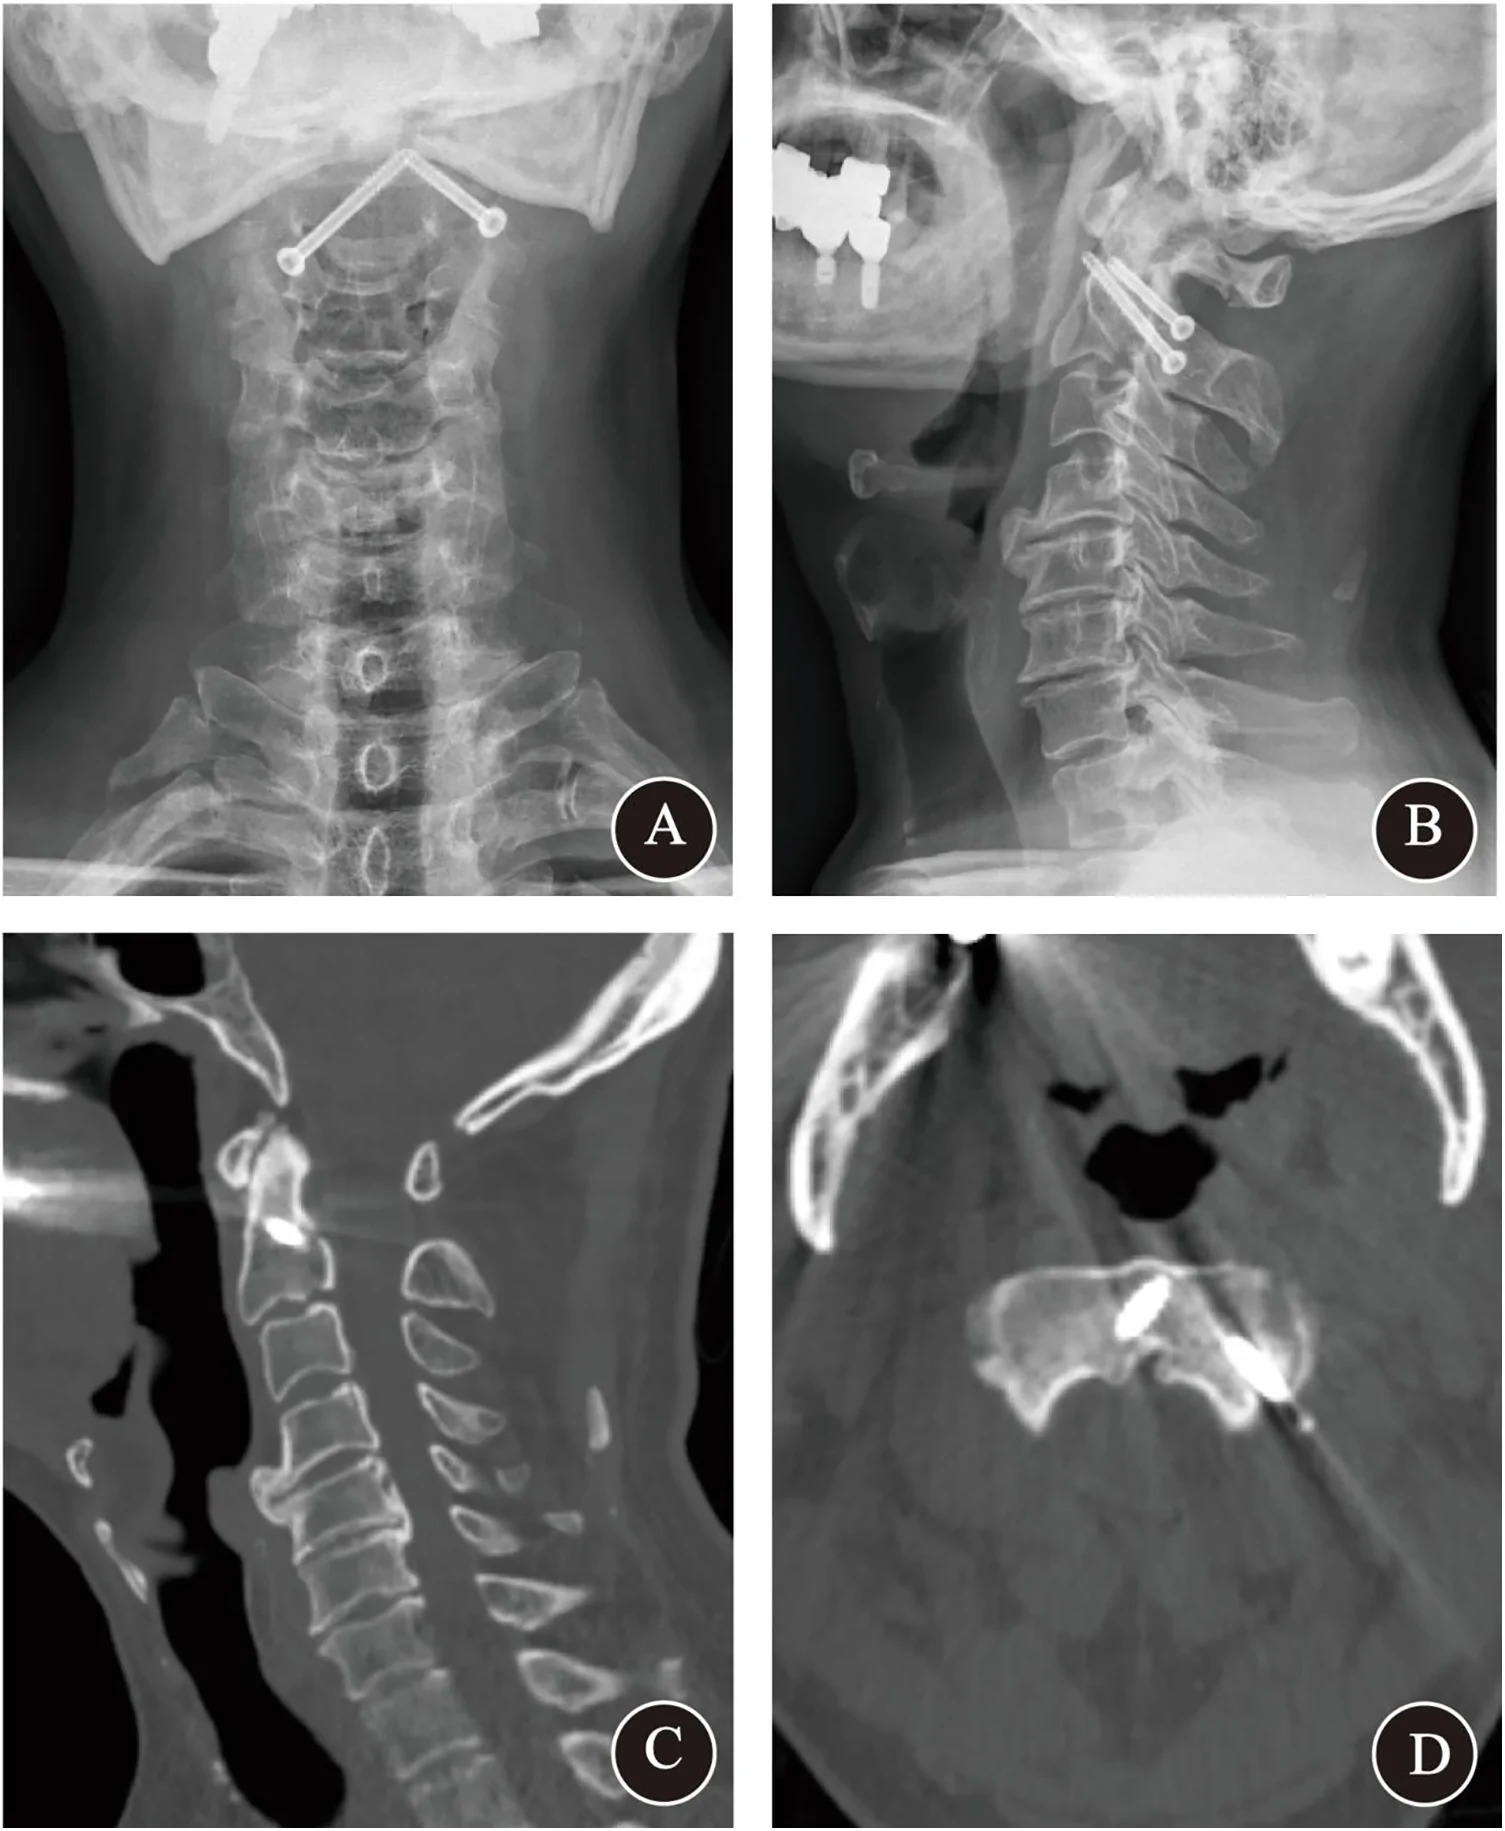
Follow-up cervical DR and CT images of the patient. (A,B) 1 month after surgery, cervical spine DR showing the proper positioning of the hollow pedicle screws. (C,D) 10 months postoperatively, cervical CT demonstrates significant fracture healing. Compared to previous examinations, the images reveal the presence of continuous bridging trabecular bone across the fracture site, accompanied by the disappearance of the fracture gap and restoration of cortical continuity.
